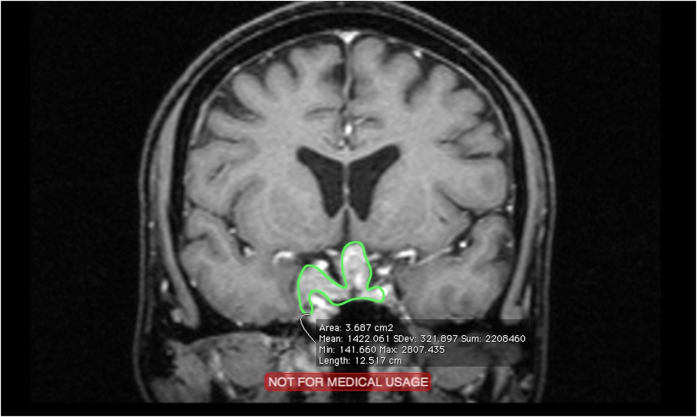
Postoperative MRI of a 55-year-old male patient revealed an irregularly shaped residual tumor with CS invasion after STR (green area).
